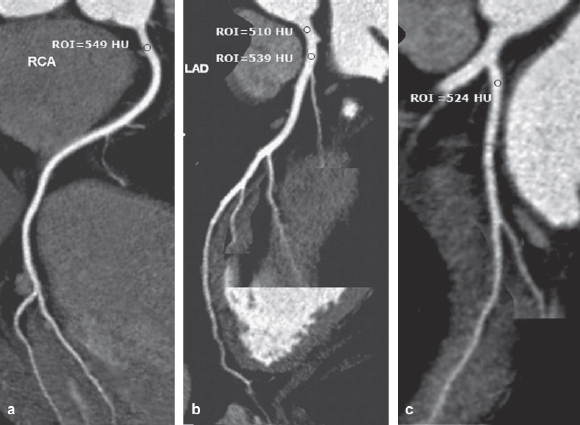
Curved planar reformation images of coronary CT angiography using iomeprol 400 are shown in a 47-year-old male. The attenuation numbers using the region of interest technique of the proximal portion of the coronary arteries were higher than 500 HU for the proximal right coronary artery (a), the left main coronary artery and the proximal left anterior descending branch (b), the proximal left circumflex artery (c). On subjective analysis, 13 segments of the coronary arteries were assessed as grade 1 (posterolateral and posterior descending branch of left circumflex artery were not found).
